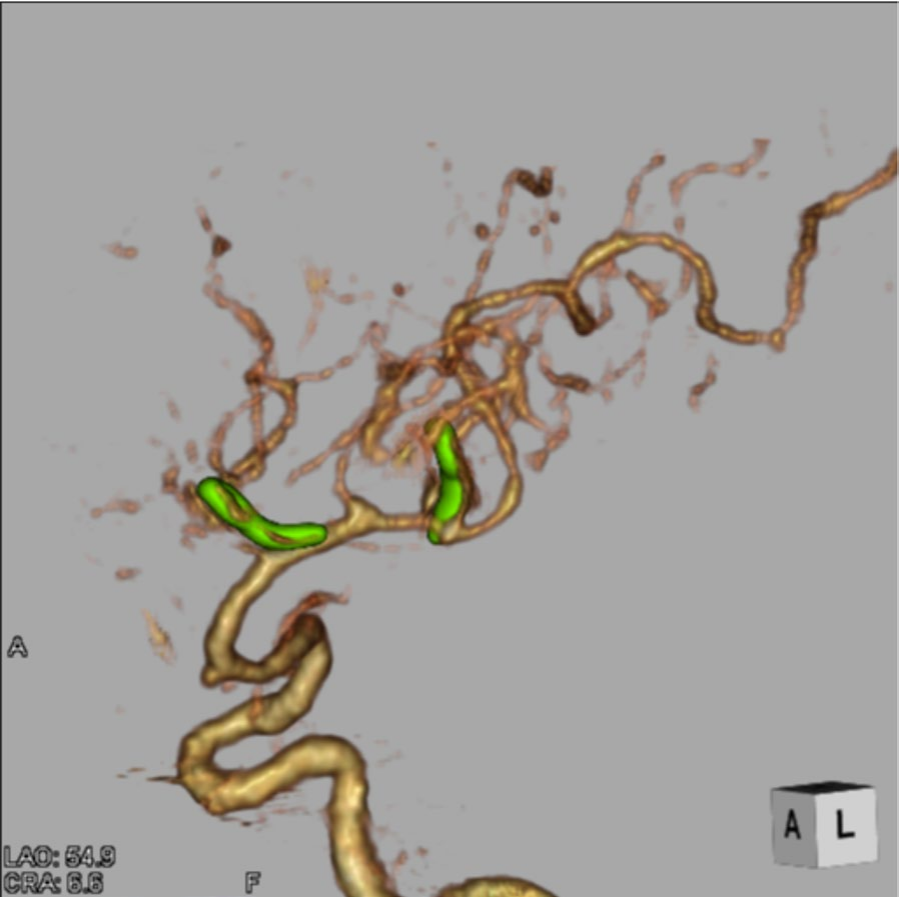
Postoperative images. The aneurysms had disappeared on computed tomography angiography after the operations
